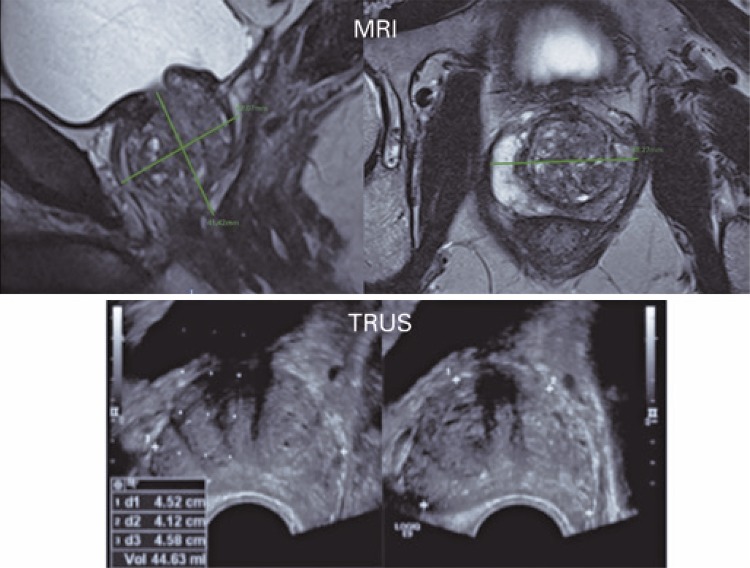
Measurements of the prostate by magnetic resonance imaging and ultrasonography on longitudinal and transverse planes MRI: magnetic resonance imaging; TRUS: transrectal ultrasonography.
